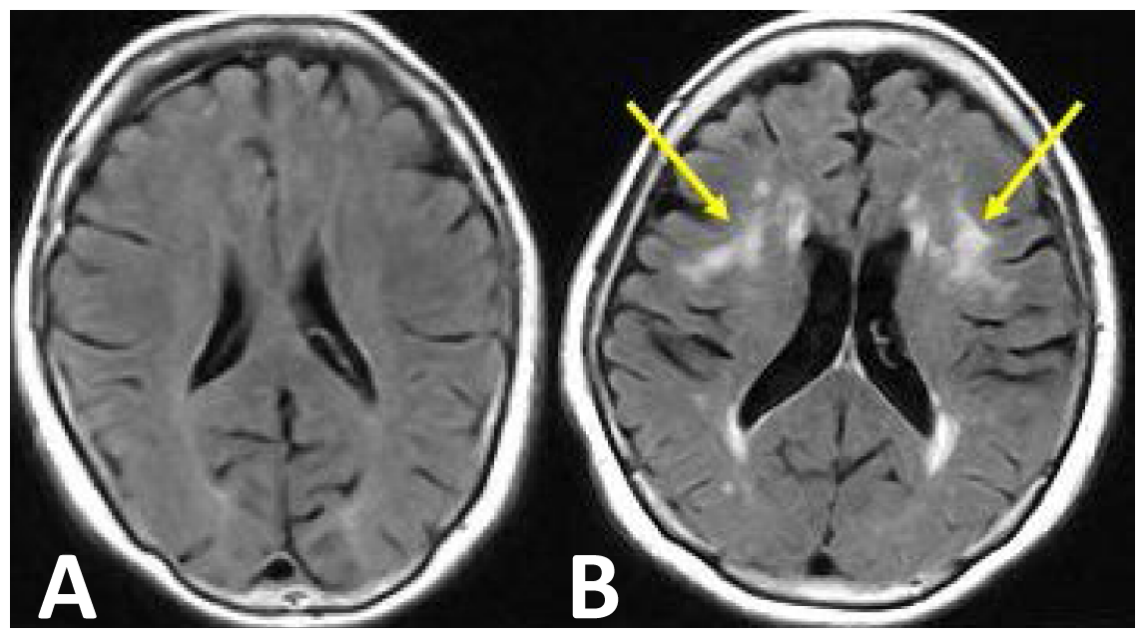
Typical MRI of G0 (right panel) and G2 (left panel; arrows indicate leukoaraiosis).
